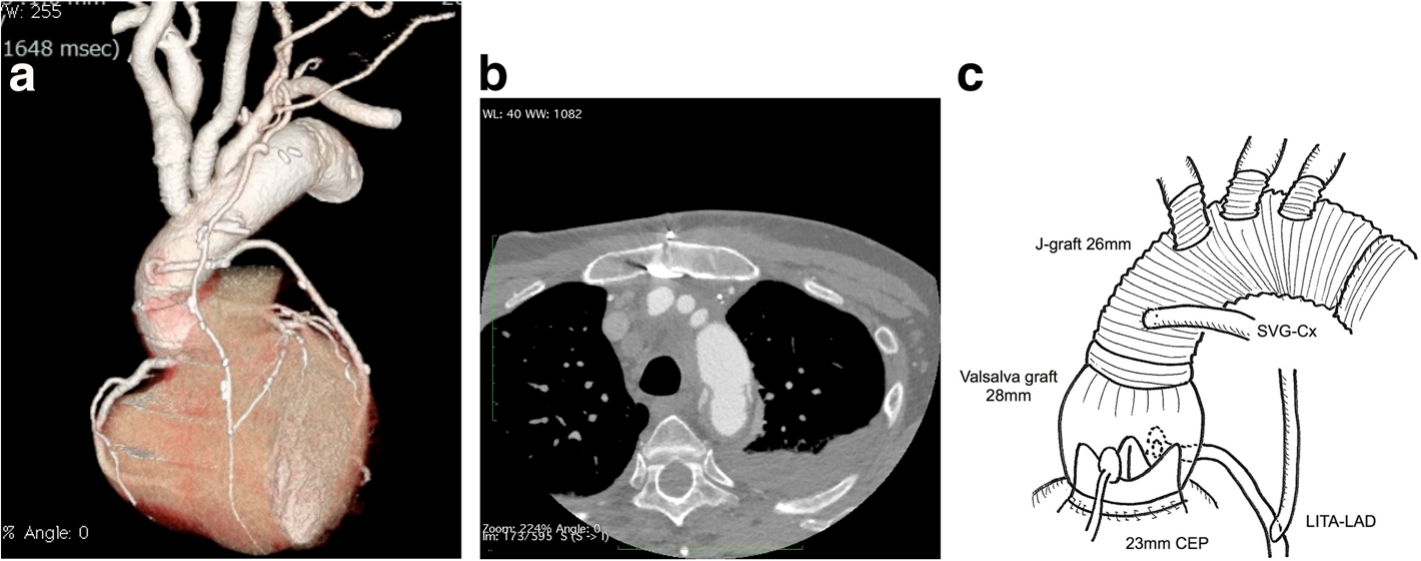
Postoperative multidetector computed tomography (MDCT) scan showing redo total arch replacement, aortic root replacement and coronary artery bypass (LITA-LAD, Ao-SVG-PL). LITA: left internal thoracic artery, LAD: left descending artery, Ao: Aorta, SVG: saphenous vein graft, PL: posterior lateral. a Synthetic image, (b) axial view, and (c) scheme of the aneurysm
